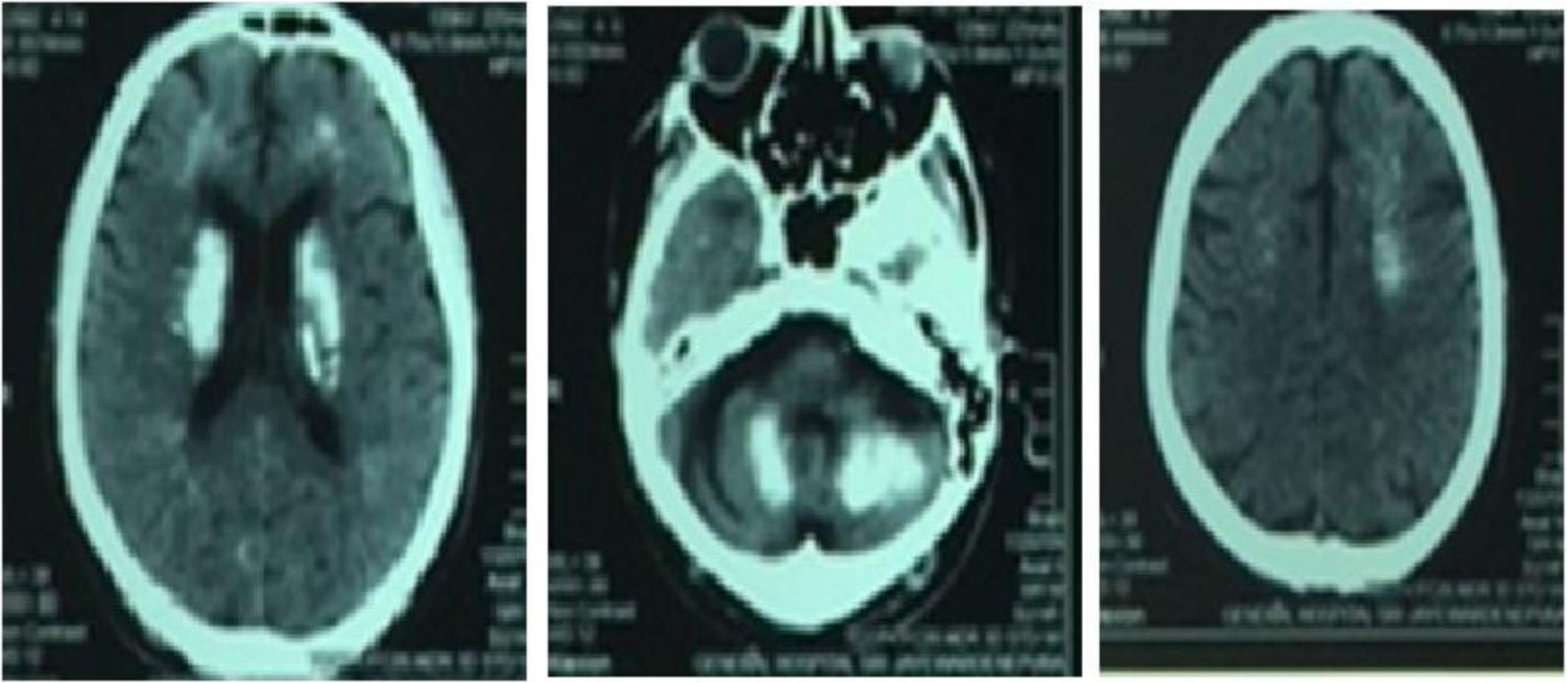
Non contrast CT scan of the brain revealing extensive cerebral calcifications in cerebral cortex and cerebellar hemispheres
